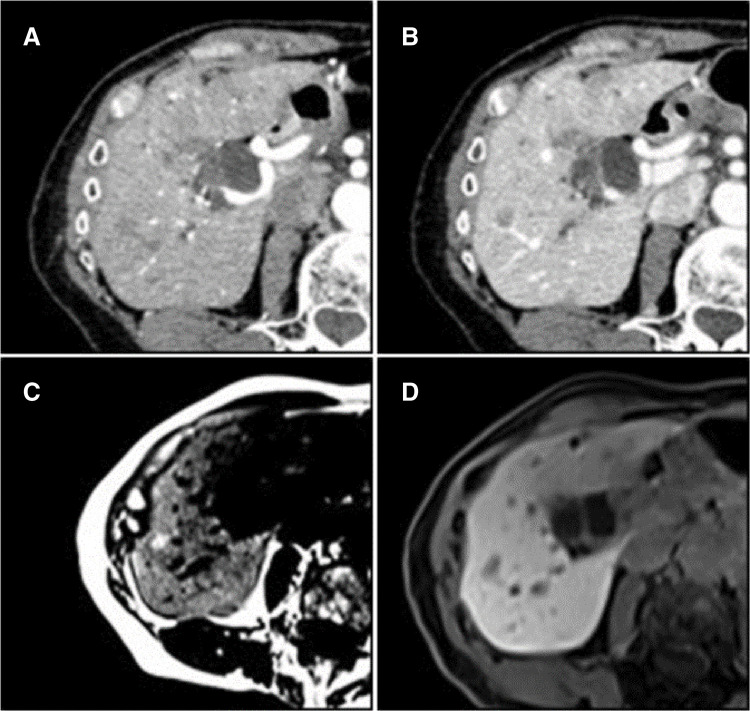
Multiple hepatic nodules. (A) Early phase of CECT. (B) Portal phase of CECT. The multiple nodules had irregular contours and were hypovascular. (C) The nodules showed high signal on MRI T1 weighted images. (D) The nodules showed mildly decreasing uptake in the hepatobiliary phase of contrast-enhanced MRI. CECT, contrast-enhanced computed tomography
